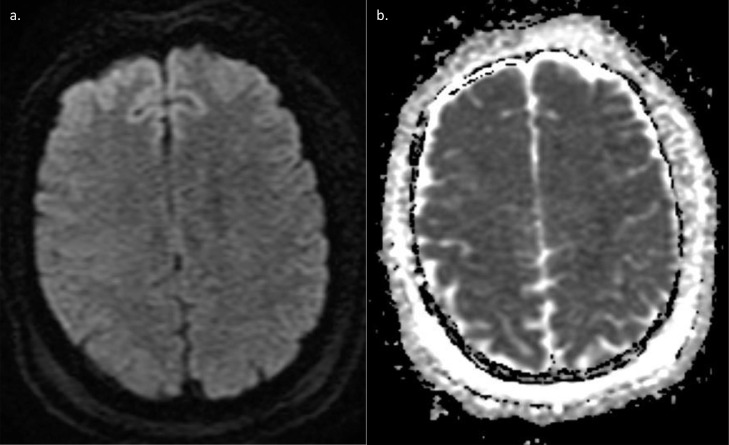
(a) DWI and (b) ADC on initial MRI examination. No evidence of restricted diffusion is seen.
